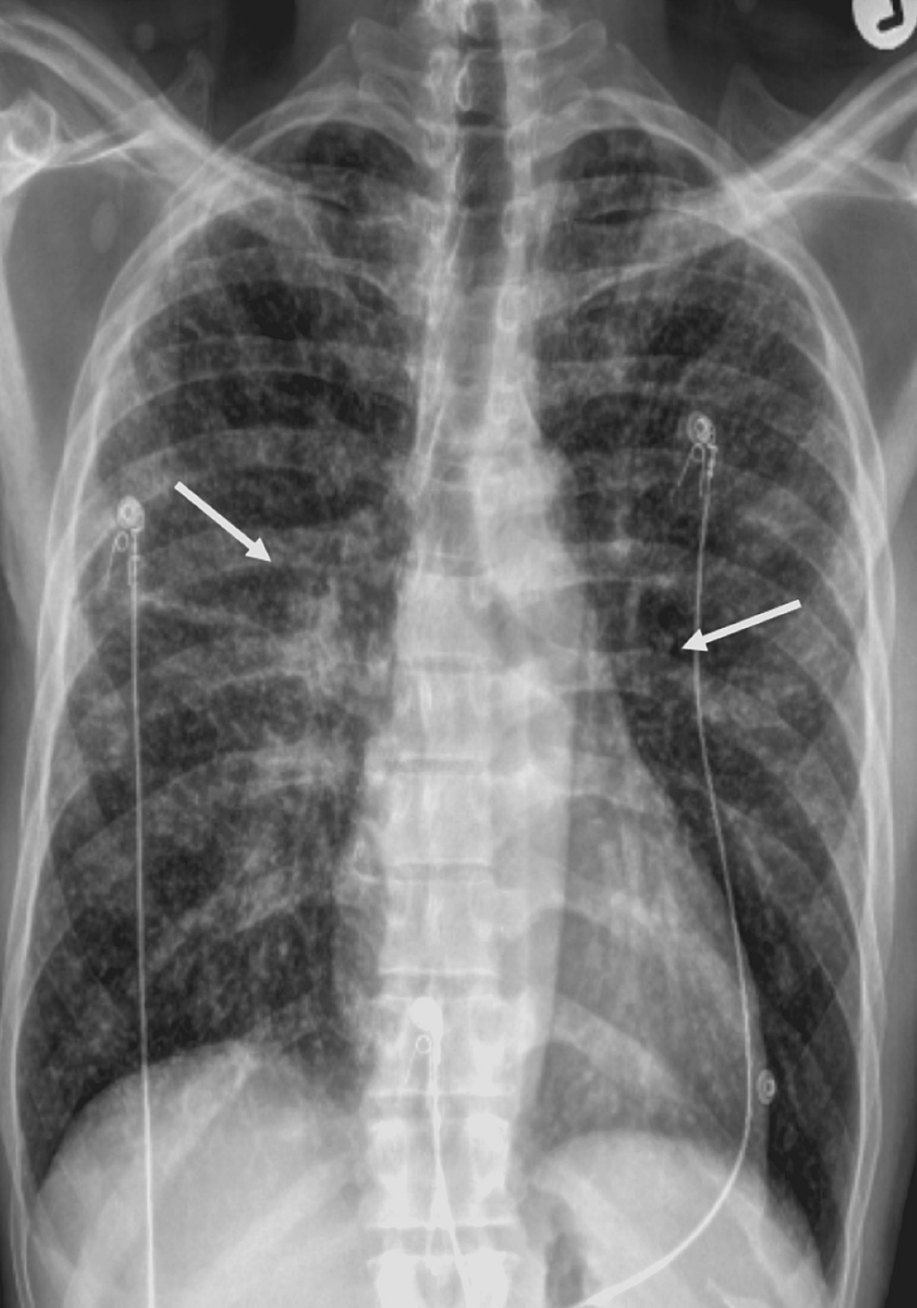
Chest X-ray (anteroposterior view) on day 12 of presentation (two days after starting prednisone therapy). White arrows show significant improvement of the bilateral hilar area.
